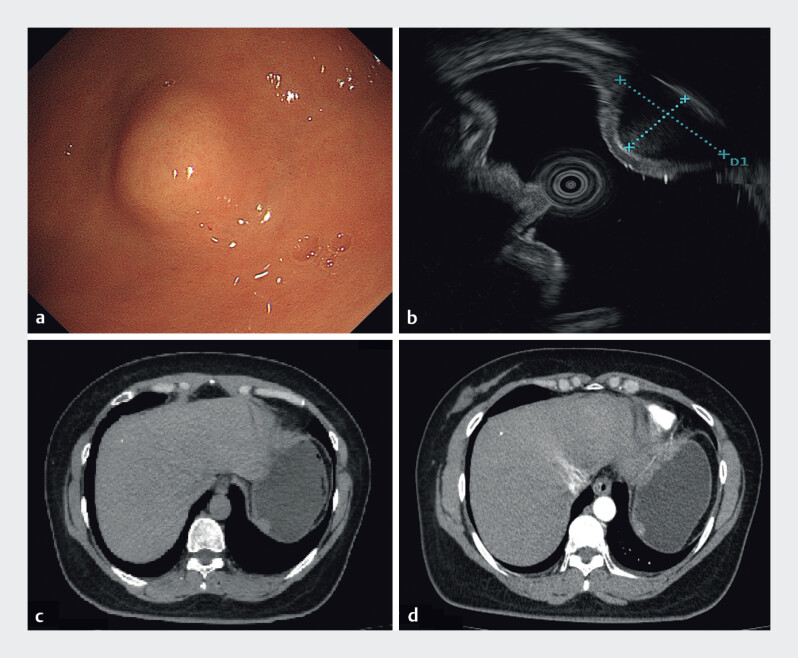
Images of the gastric fundus lesion obtained via white-light gastroscopy, endoscopic ultrasound (EUS), and abdominal pain and contrast-enhanced CT.
